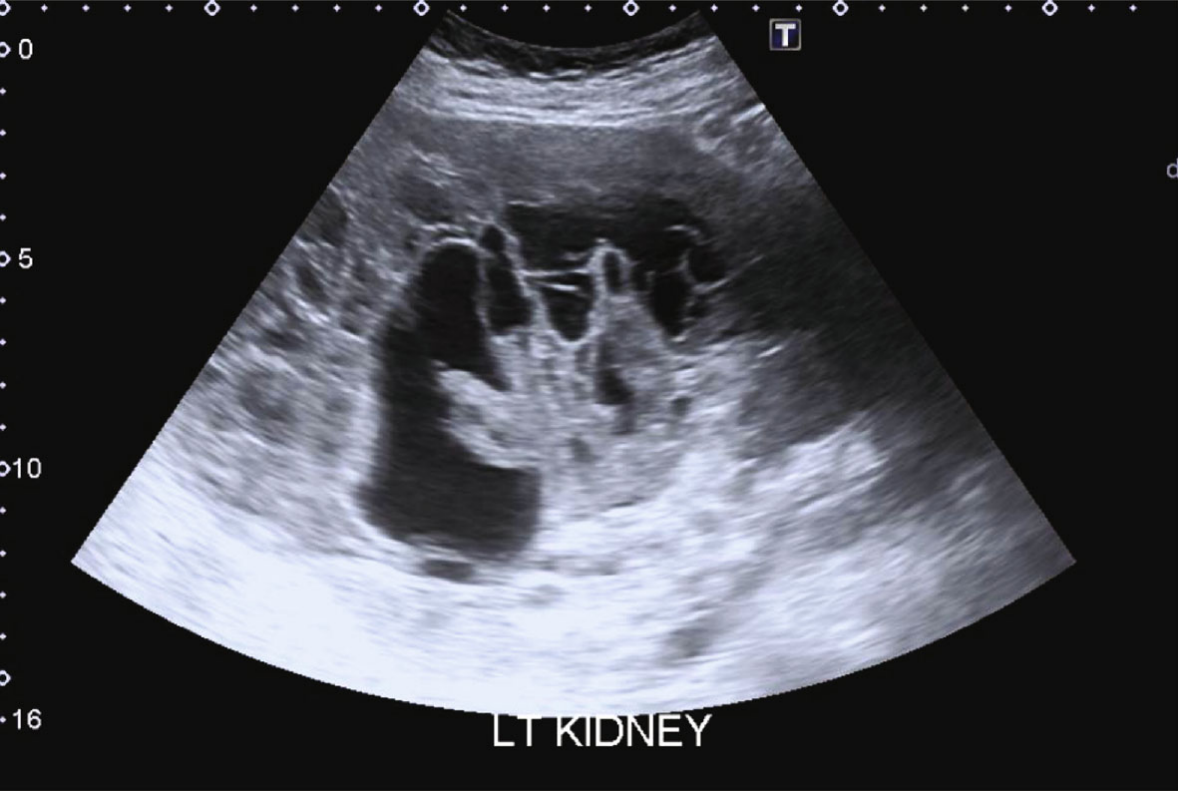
Ultrasound showing malignant looking left renal mass with mixed solid and cystic component.
